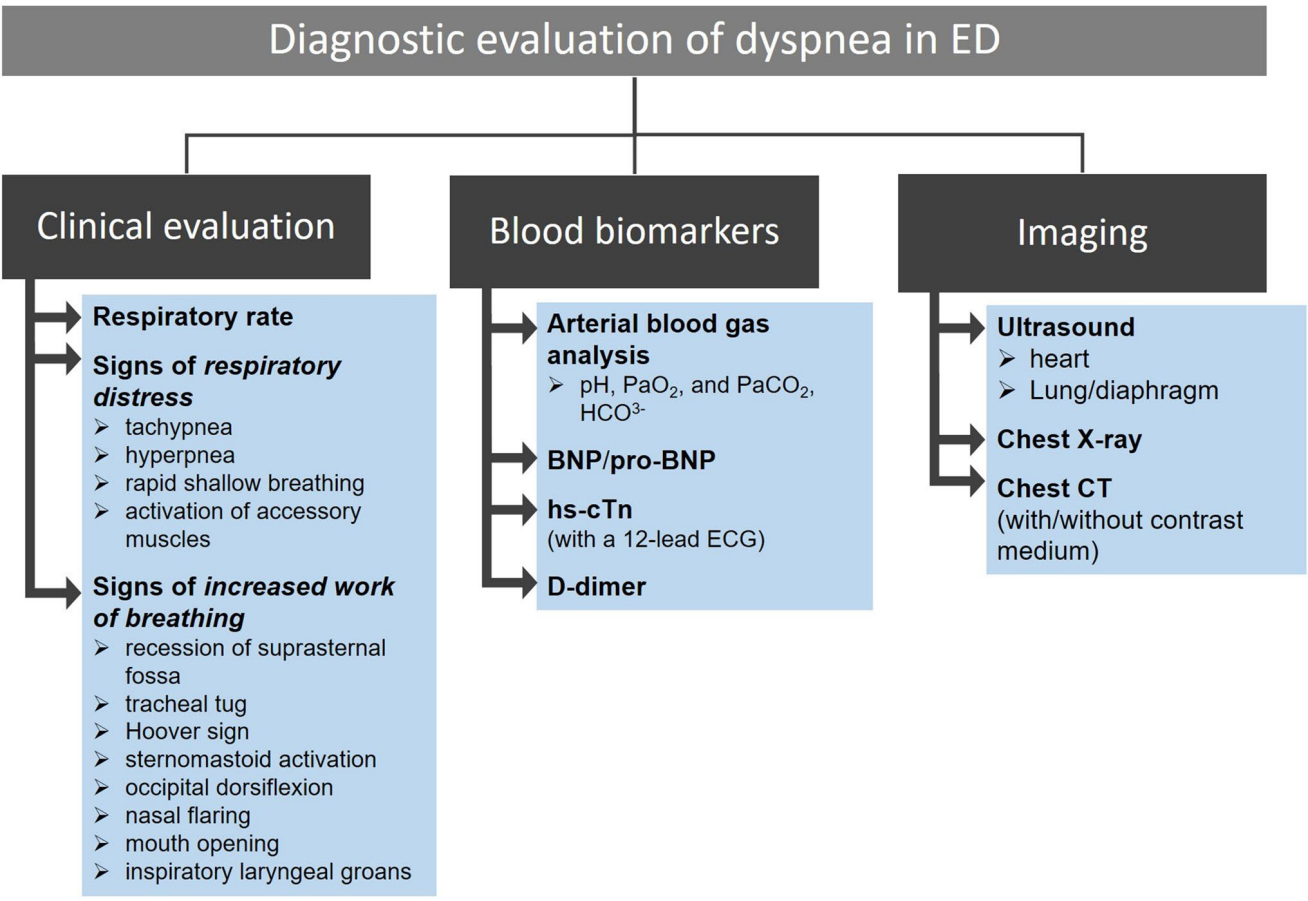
Summary of the diagnostic workup in patients with acute dyspnea presenting at the emergency department. BNP: B type natriuretic peptide; NT-proBNP: N-terminal pro B-type natriuretic peptide; hs-cTn: high sensitivity cardiac troponin; CT: computed tomography; PaO_2_: arterial partial pressure of oxygen; PaCO_2_: arterial partial pressure of carbon dioxide; HCO^3−^: bicarbonate
